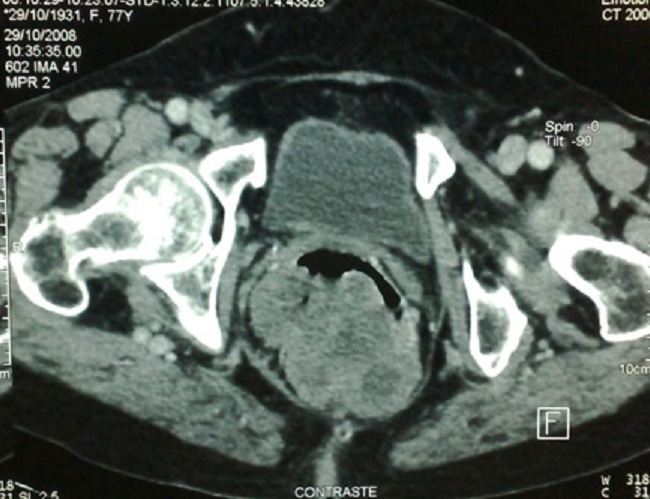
Pelvic CT scan showing the rectal lesion
